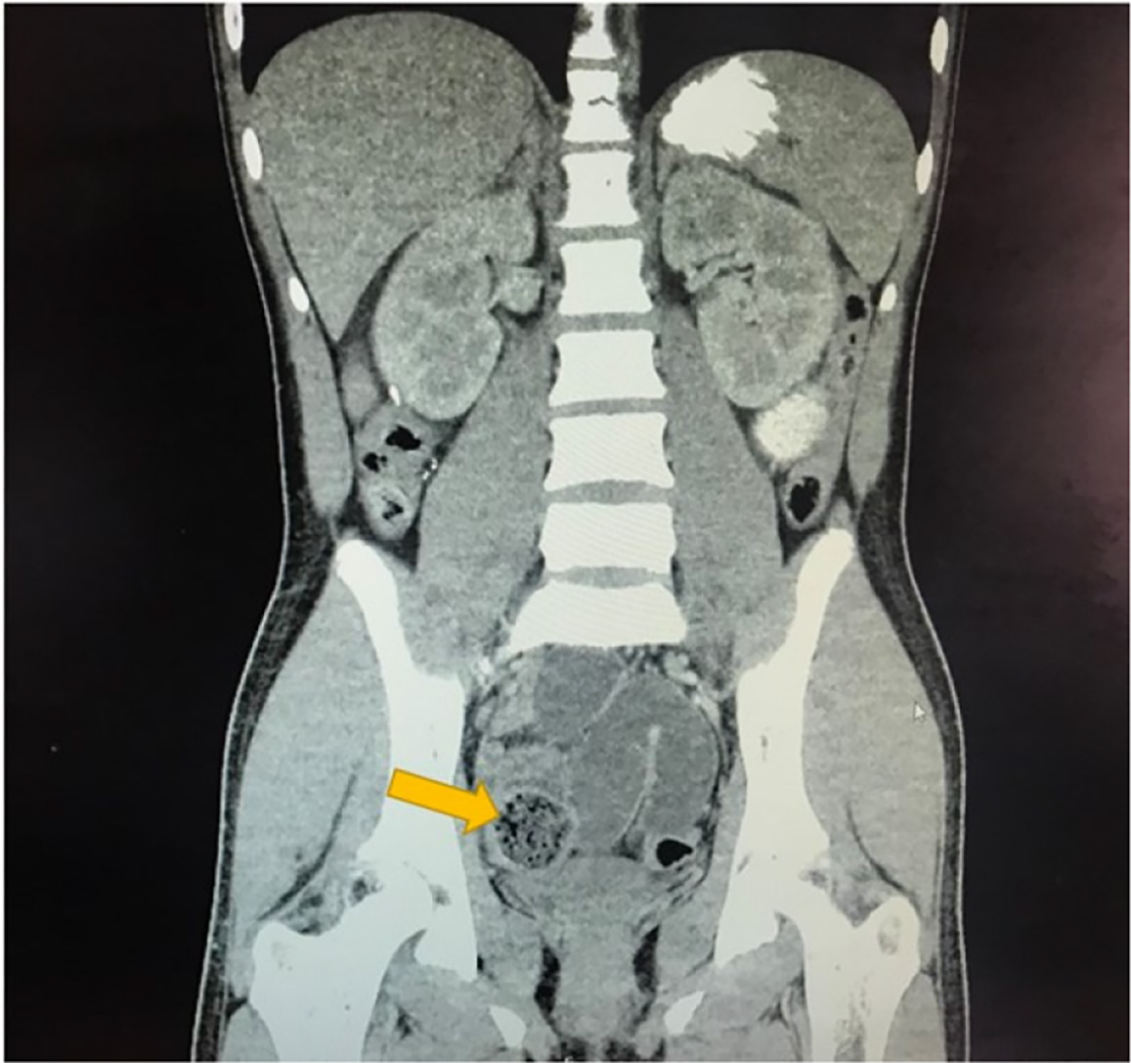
CT scan of the abdomen and pelvis with contrast showing a focus of fecalized small bowel contents (arrow).
